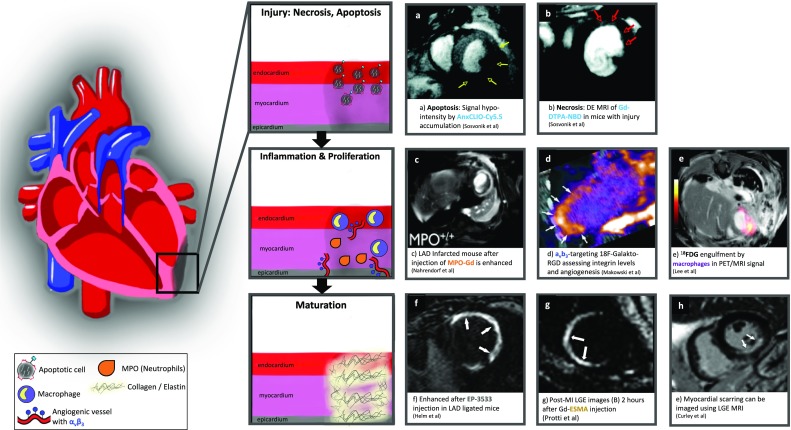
Representative examples of targeted and non-targeted contrast agents developed to evaluate biological alterations after myocardial infarction using MRI and PET
